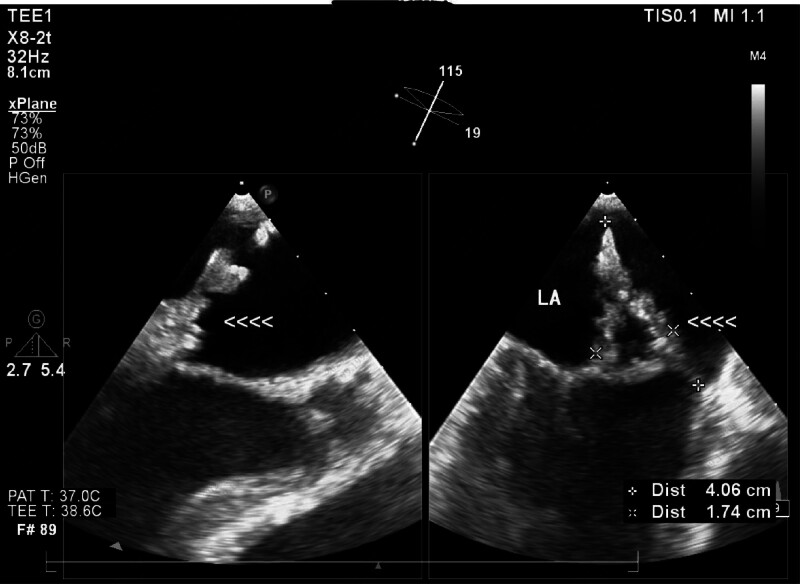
Mitral valve vegetations visible on transesophageal echocardiography.
